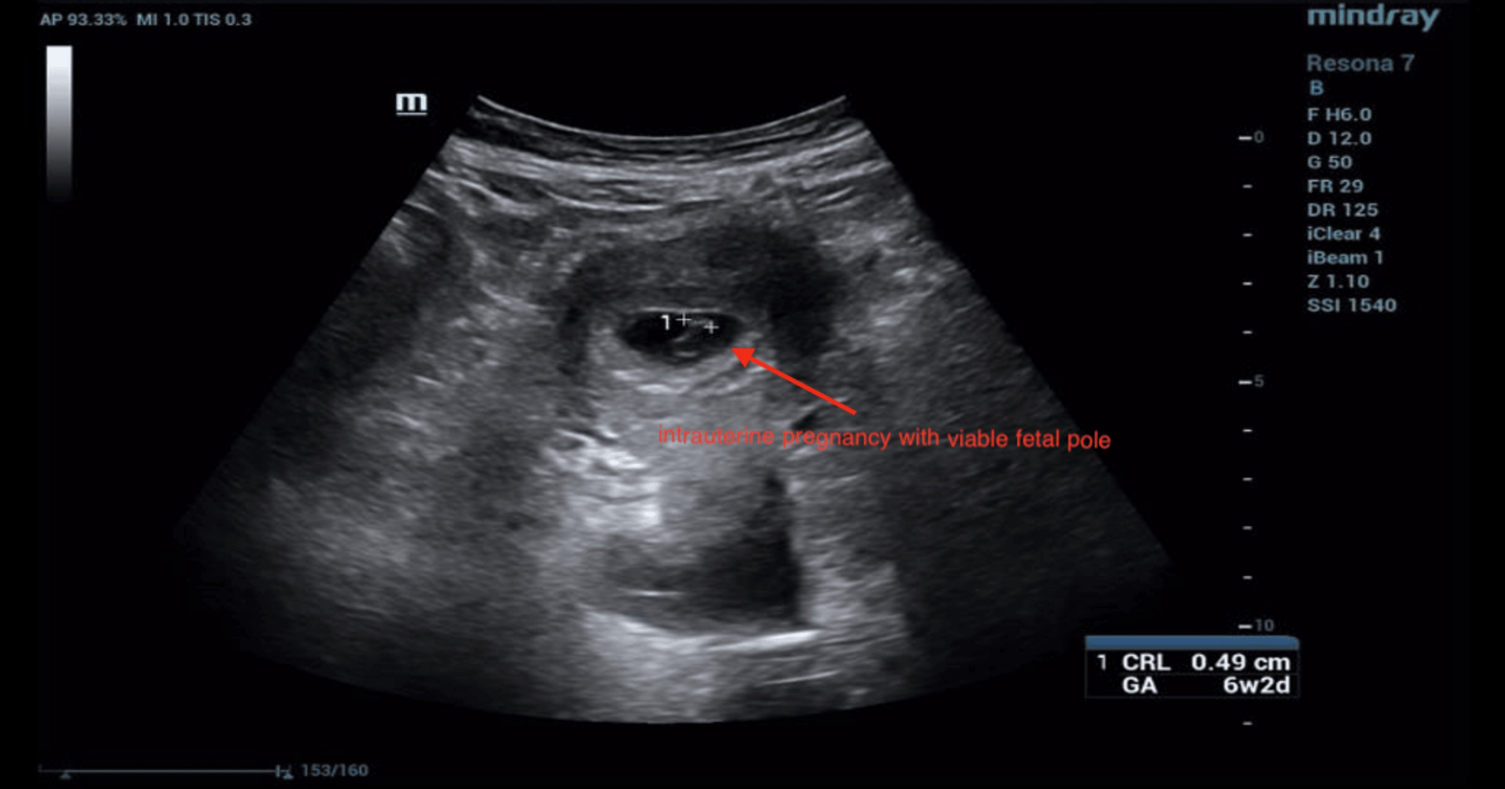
Transabdominal ultrasonography image of uterus shows a single gestational sac seen in the uterine cavity containing single viable fetal pole, corresponding to six weeks and two days, with a crown-rump length of 0.49 cm.
